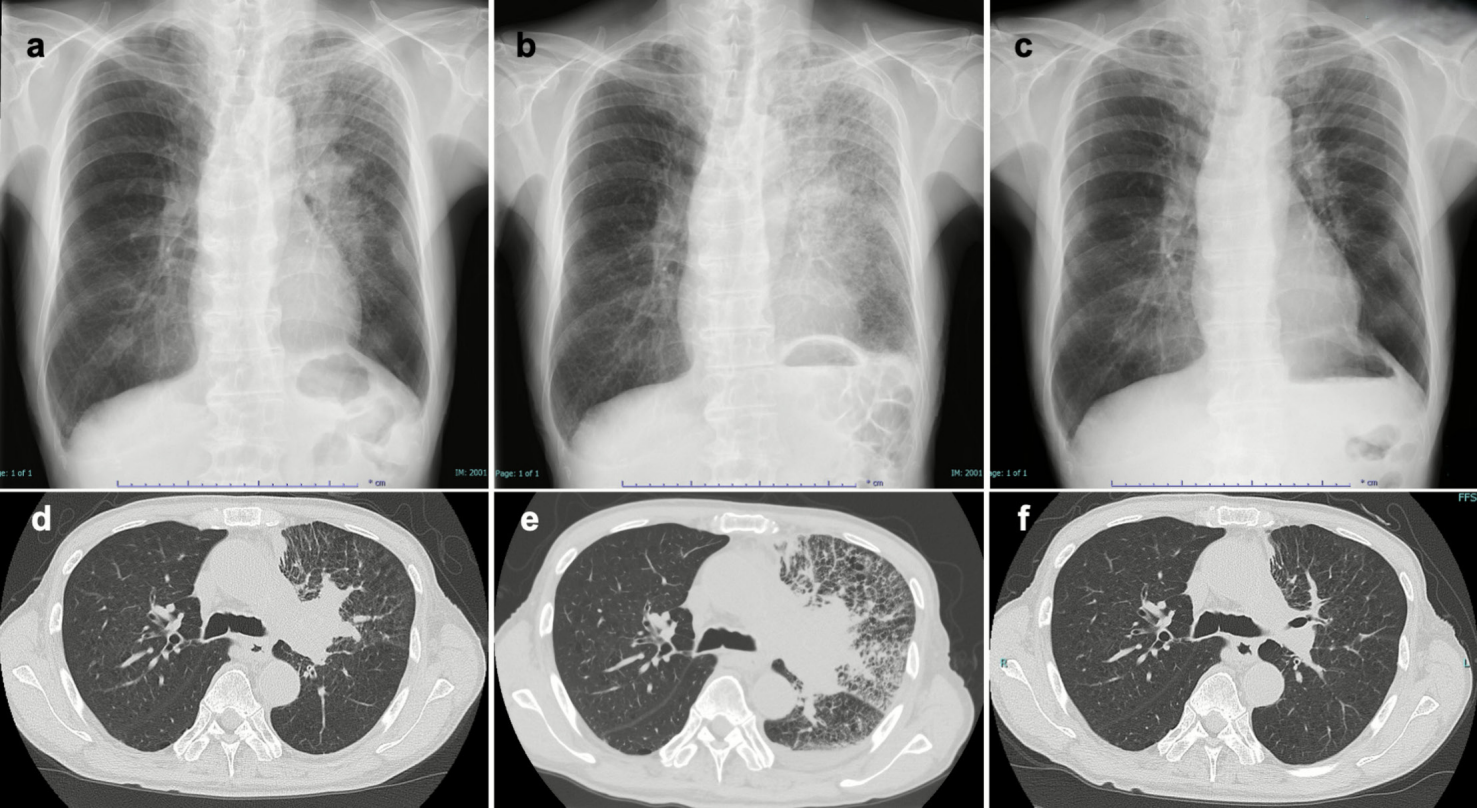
Serial chest imaging before and after tarlatamab administration. (A) Baseline chest x‐ray showing a mass in the left upper lung field; (B) chest x‐ray on Day 3 showing extensive ground‐glass opacities and infiltrates in the left lung; (C) chest x‐ray on Day 28 after the first dose showing resolution of infiltrates and a left hilar mass smaller than at baseline. Chest CT images obtained at baseline (D), on Day 3 (E), and on Day 38 (F).
